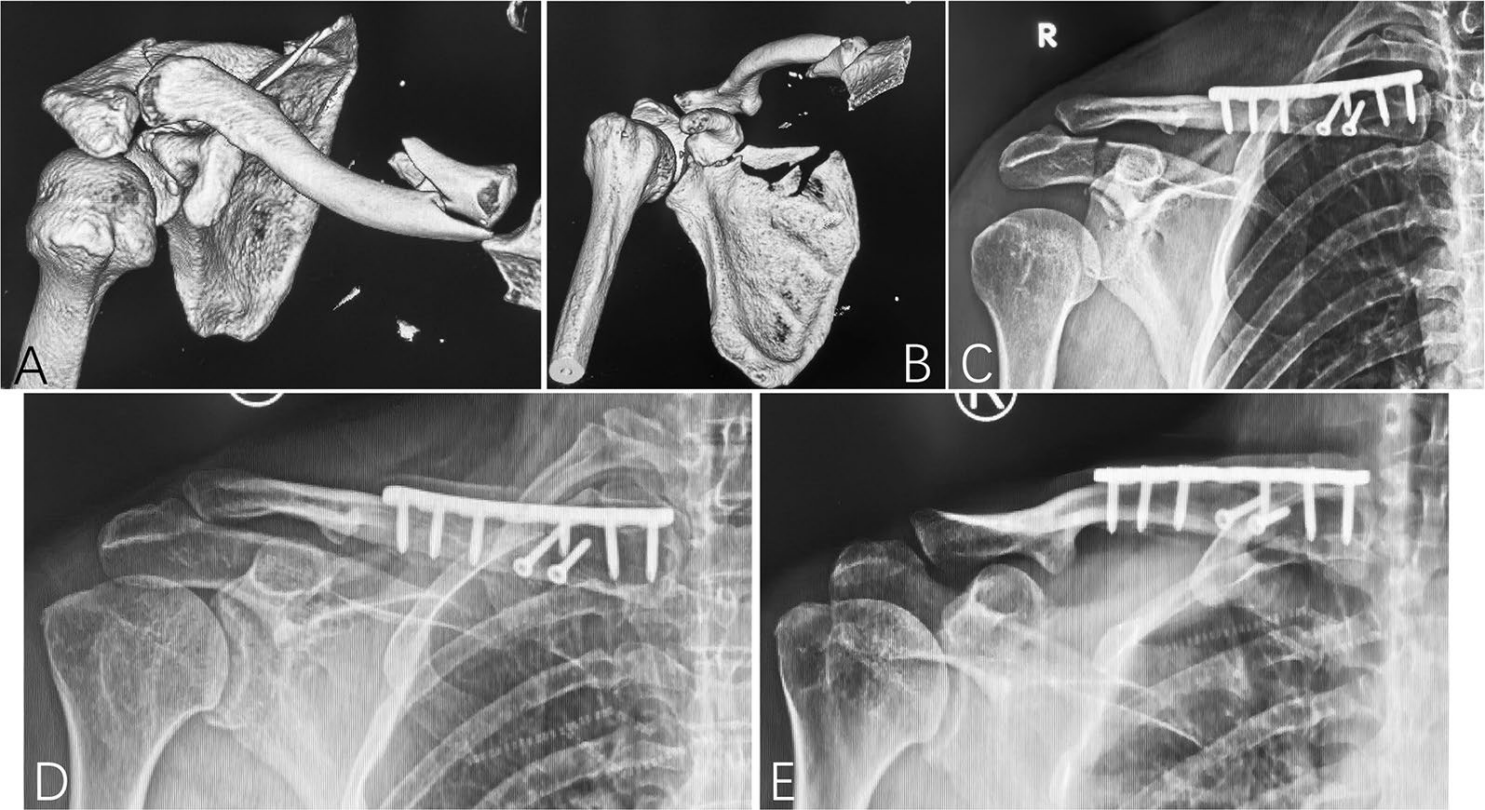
A 41-year-old male worker (patient 11) was injured by falling glass while at work. A, B He sustained a displaced medial clavicle fracture, an ipsilateral type V coracoid process fracture, an ipsilateral acromion fracture, transient brachial plexus injury, and chest injury. C The medial clavicle fracture was fixed with plating. D, E X-rays taken 24 months postoperatively showed solid bone union of the clavicle, coracoid process, and acromion
